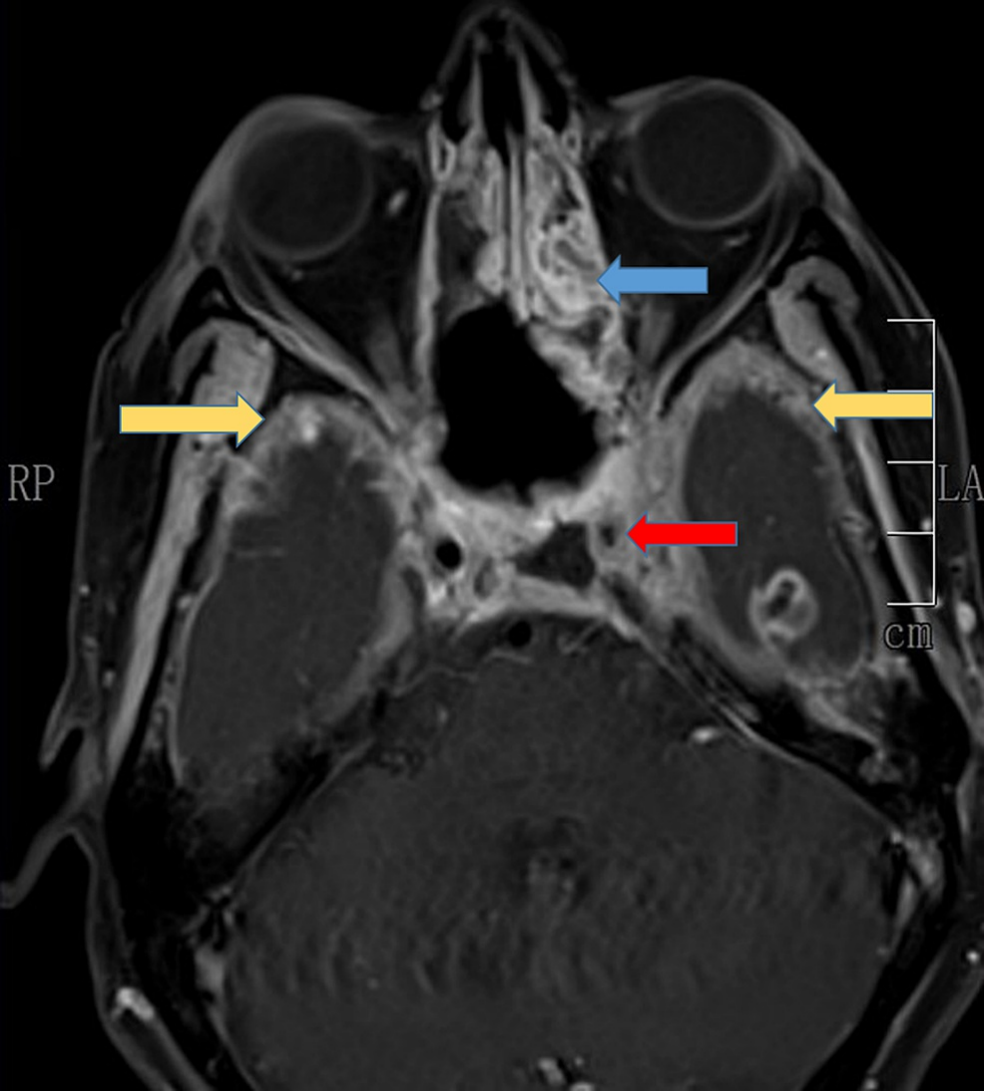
MRI brain, axial view showing partial thrombus in the cavernous portion of ICA (red arrow), left ethmoidal sinusitis (blue arrow), and meningeal enhancement over temporal lobes bilaterally (yellow arrow) ICA: internal carotid artery
